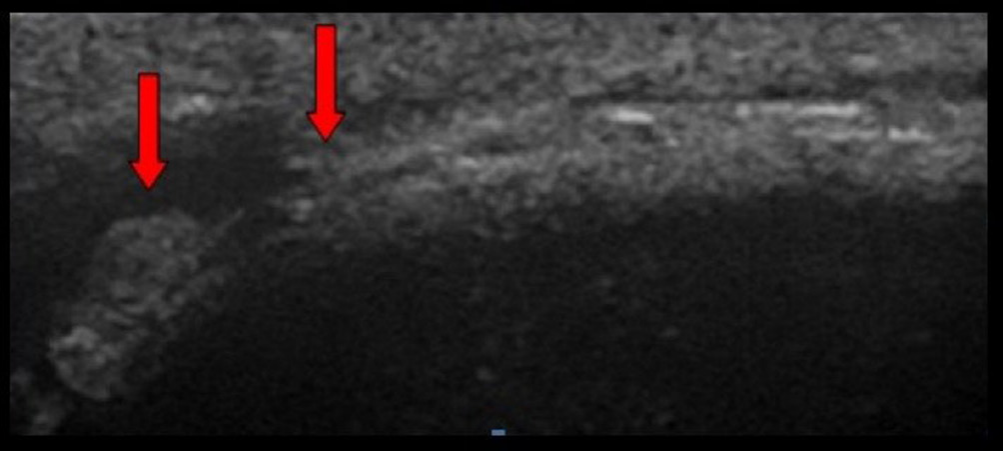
Ultrasound image showing thrombosis of the superficial dorsal vein of the penis (arrows).
